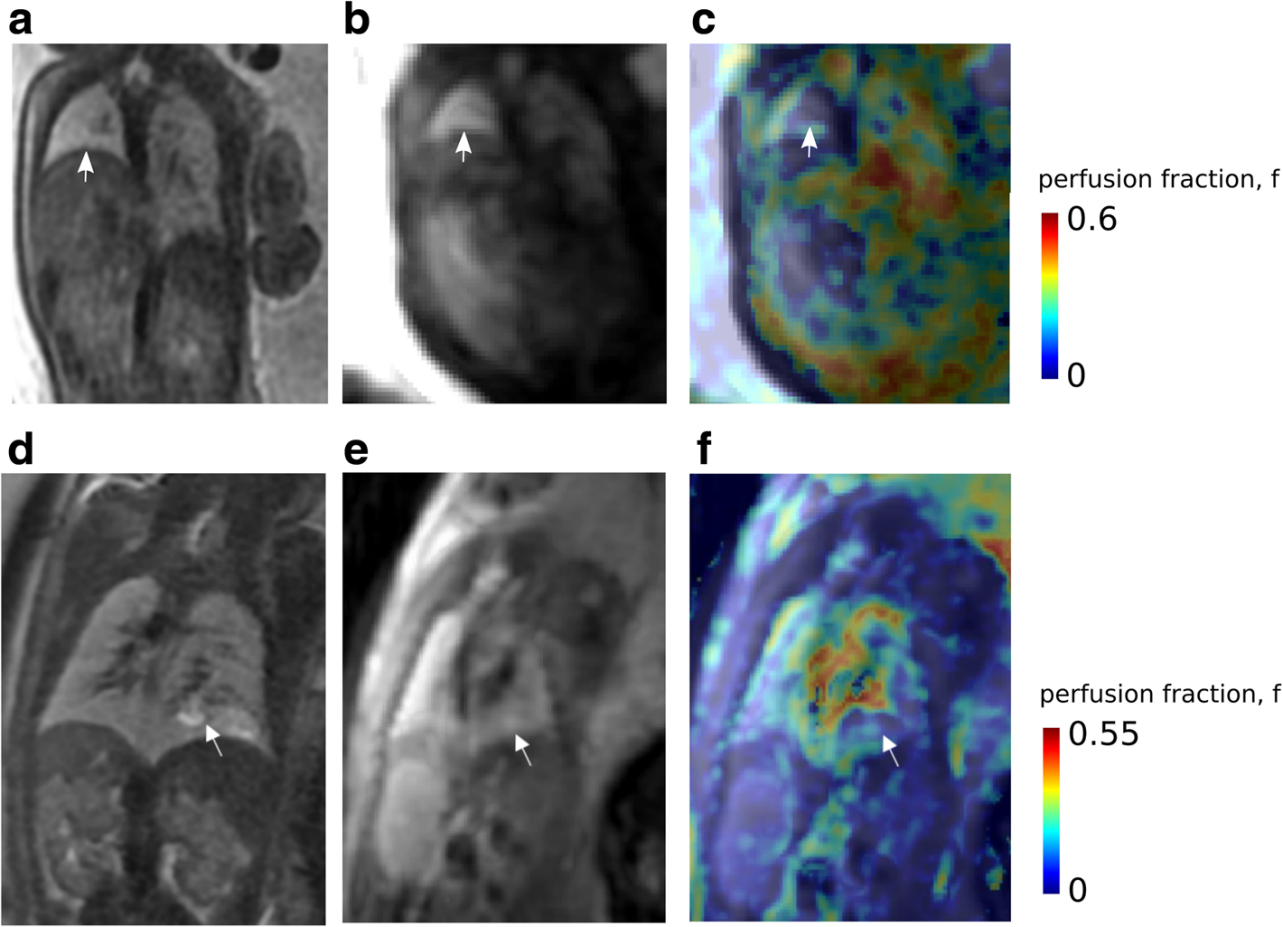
a–c Congenital diaphragmatic hernia: hypoplastic left lung (*arrow*); normal right lung. a T2-weighted coronal image. b DWI, b-factor = 0 image. c IVIM perfusion fraction map showing decreased microvascular perfusion fraction in the hypoplastic left lung. d–f Congenital cystic adenomatoid formation of the right lung. d Coronal T2-weighted image showing a hyperintense abnormality at the inferior lobe of the right lung. e DWI, b-factor = 0 image. f IVIM perfusion fraction map showing a demarcated zone of decreased microvascular perfusion fraction in the affected region of the inferior lobe (*arrow*)
